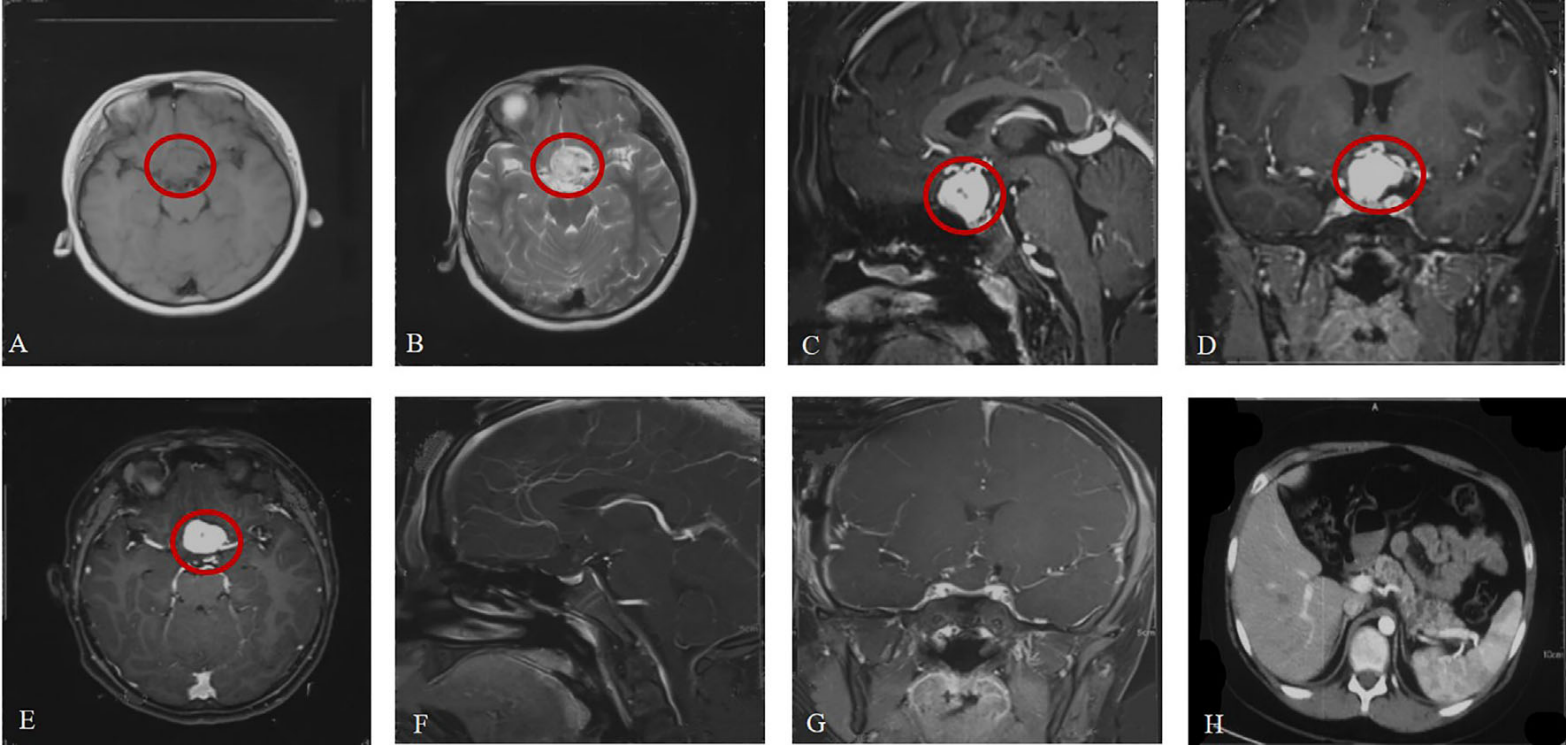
Brain MRI at admission (A–E). (A, B) Axial view (weighted sequence in T1, T2). (C–E) Sagittal, coronal, axial view after contrast injection, with the tumor marked. (F, G) Brain MRI after surgery. (H) Pancreatic cysts.
